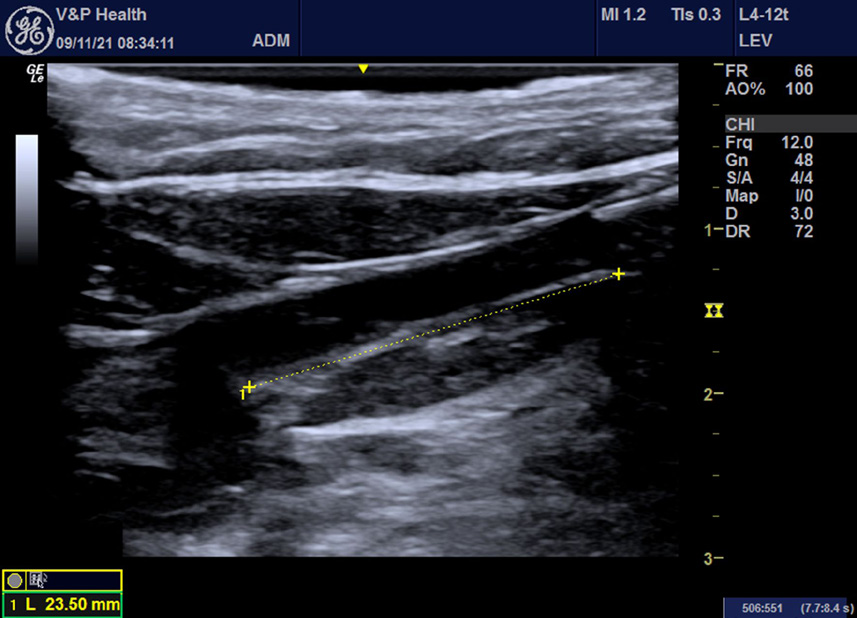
Longitudinal color Doppler ultrasound image in B-mode showing the distance from the fiber tip to the saphenofemoral junction.8 - Venous tumescence is obtained using 0.9% saline, working in the foot-to-head direction, until reaching the SFJ (Figure 3);
